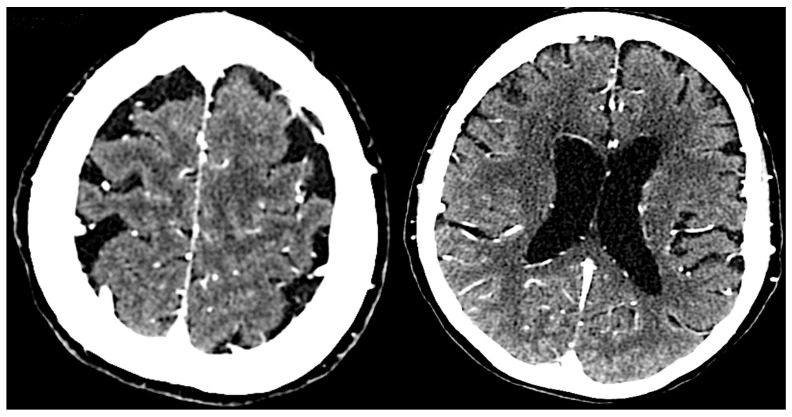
The brain CT scan shows cerebral atrophy with slight ventricular enlargement.
